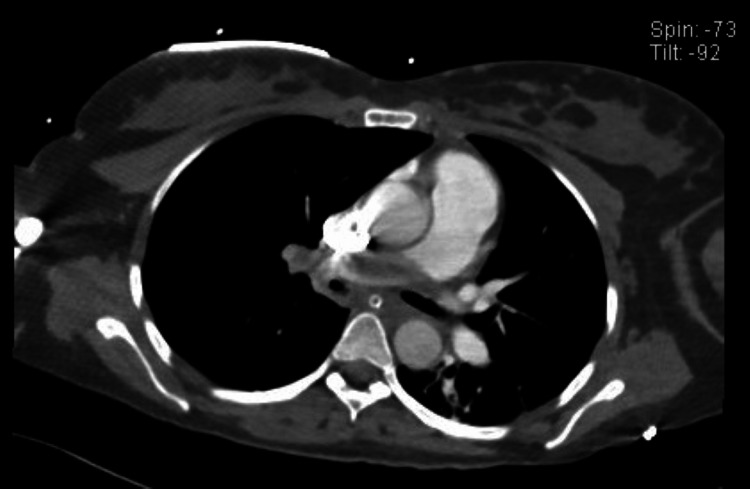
Computed tomography pulmonary angiogram showing a large filling defect at the pulmonary trunk, completely obliterating the right pulmonary artery extending to the proximal left pulmonary artery
